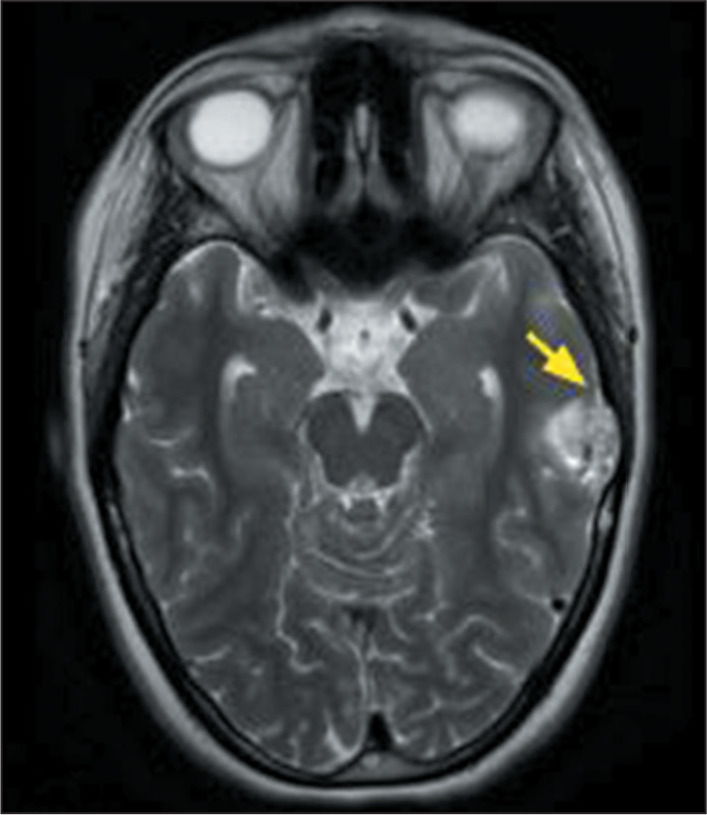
MRI examination of a 20-year-old female patient diagnosed with a
